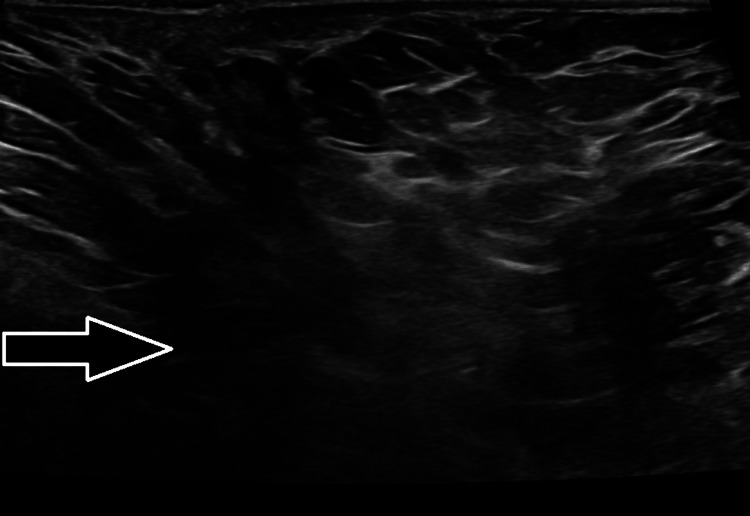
Ultrasound scan at initial presentation (arrow).
